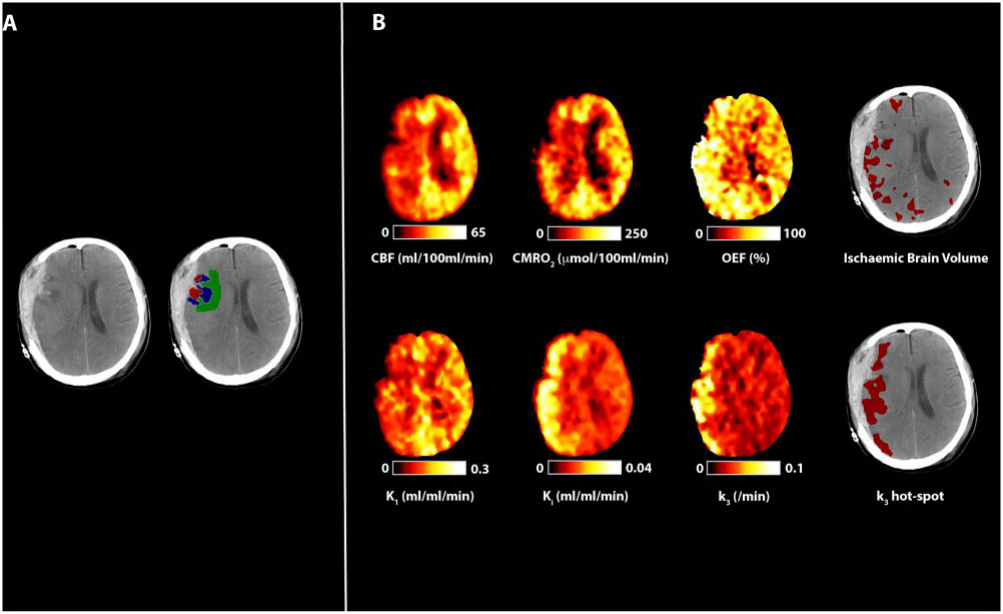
Evidence of cerebral ischaemia and hyperglycolysis. CT, CBF, CMRO_2_, OEF, and ^18^F-FDG kinetic parameters obtained in a 40-year-old female within 24 h of severe TBI resulting from a fall. Imaging was obtained following evacuation of a subdural haematoma. Plasma glucose during PET was 3.7 mmol/l. (A) CT demonstrates residual subdural blood with minimal midline shift and underlying haemorrhagic contusions. Regions of interest are defined for haemorrhagic lesion (core, red), hypodense tissue (penumbra, blue), and a 1 cm border zone of normal-appearing tissue (peri-penumbra, green). (B) Co-registered parametric maps of CBF, CMRO_2_, OEF, K_1_, K_i_ and k_3_. The regions with a critical increase in OEF above the individually calculated threshold (ischaemic brain volume) and increased phosphorylation activity (k_3_ ‘hotspot’) are both shown in red overlying the CT scan. The ischaemic brain volume and k_3_ hotspot volume were 96 ml and 174 ml, respectively, and the volume of overlap between these two tissue classes in this patient was 38 ml.
